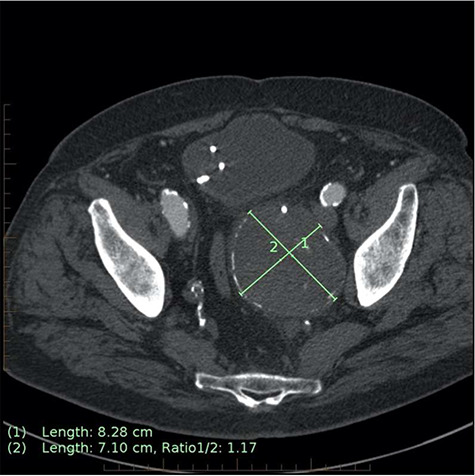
Axial CT image on 6-month follow-up demonstrating expansion of the IIAA by 7 mm, now measuring 83 × 71 mm axially (previously 76 × 62 mm).
